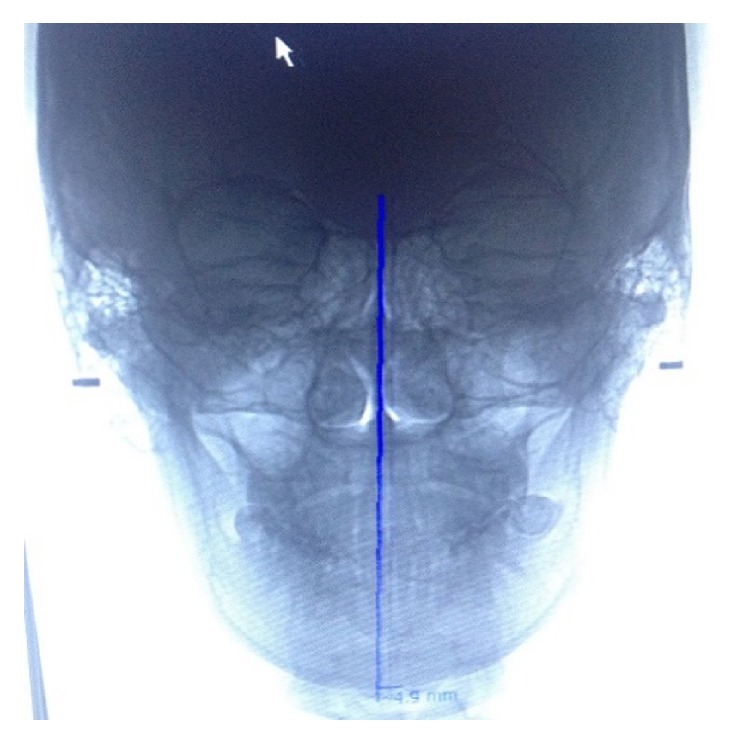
Menton deviation to MSR in PA cephalogram digital radiograph.
